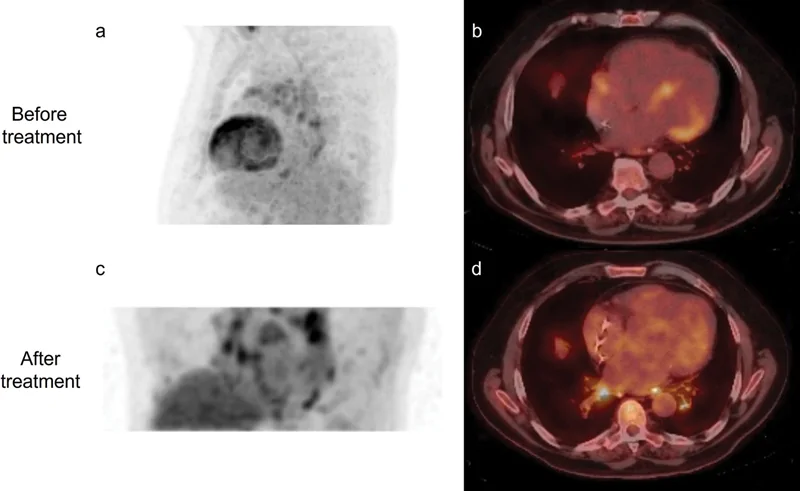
Serial fluorine-18 fluorodeoxyglucose (FDG) positron emission tomography/computed tomography (PET/CT) imaging demonstrating treatment response in cardiac sarcoidosis. (
